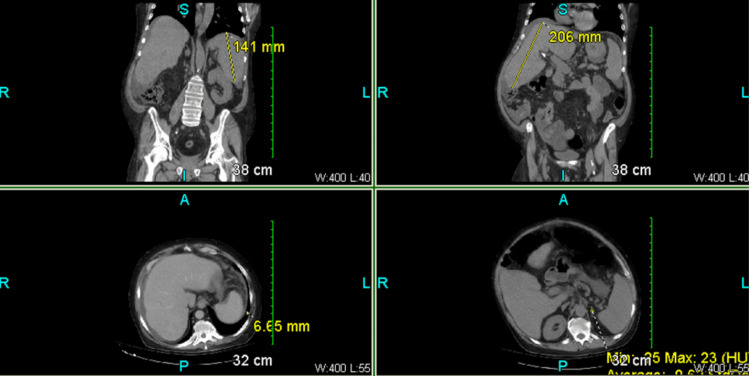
Case one: abdominal CT images showing a 7 mm left lung nodule, cirrhotic liver, ascites, dilated small bowel loops, and decompressed distal bowel
